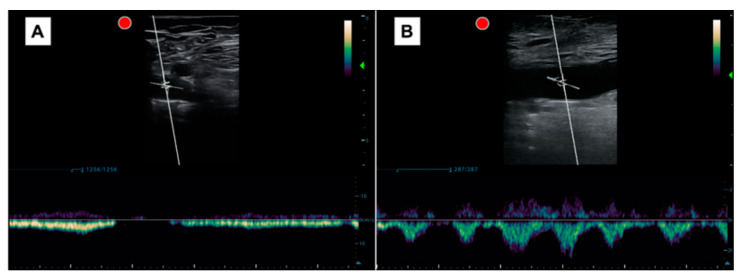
(A) Pulsed Doppler spectral waveform at the right CFV with a normal pattern of respiratory modulation. The flow is directed towards the heart in the expiratory phase and stops in the inspiratory phase. (B) Pulsed Doppler spectral waveform at the right CFV with an anormal pattern with cardiac modulation. Systolic and diastolic waves were clearly identified whether or not retrograde flow was present during spontaneous inspiration. Red dot: indicates the location of the probe marker.
